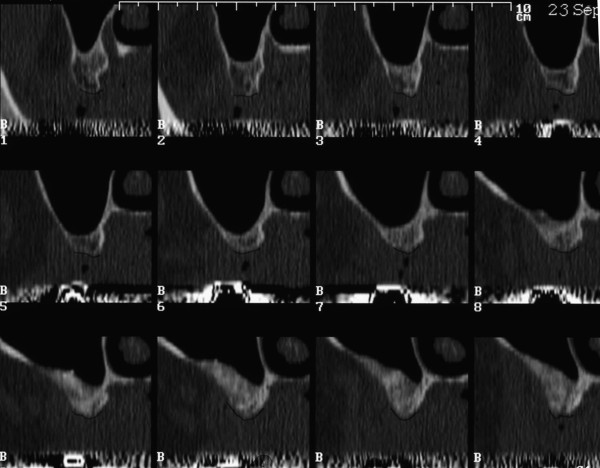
Dental-CT: Layers B1 – B12 = regio 18 – 14; Distance between the layers: 2.5 mm (1:1).
